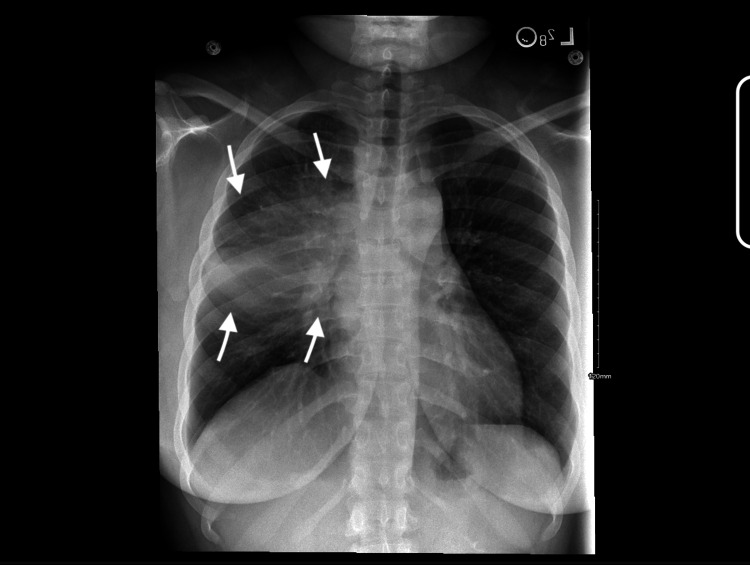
First chest X-ray performed during initial hospitalization prompting a workup for pneumonia
